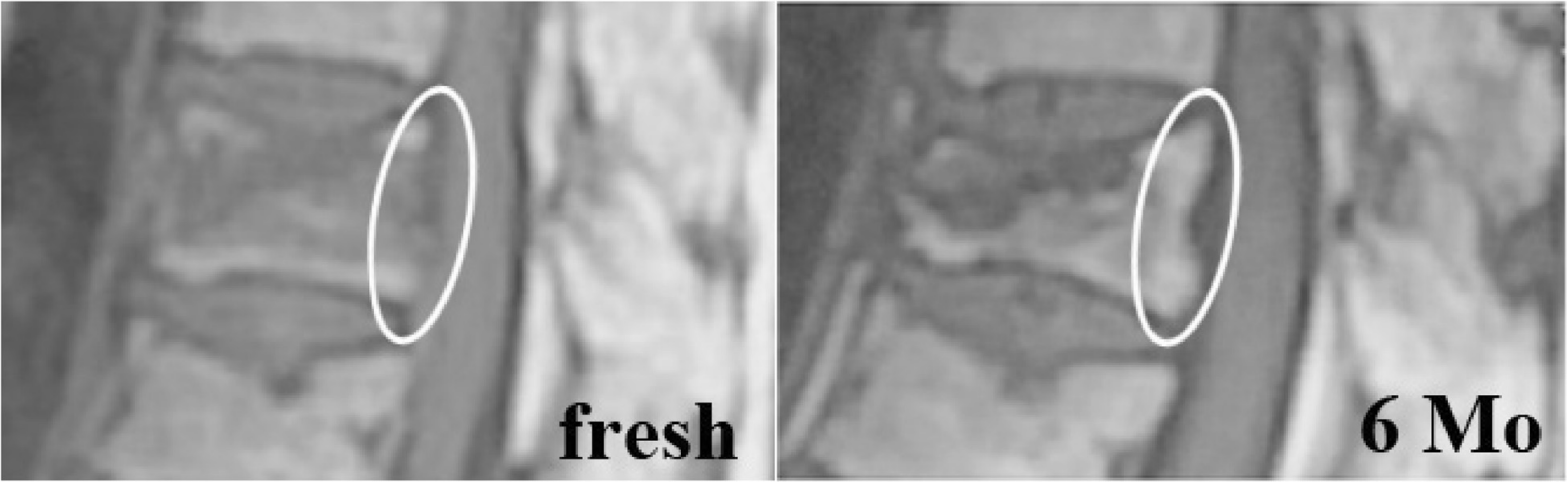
Representative image of chronological intensity change at posterior wall on T1-weighted magnetic resonance image. Intensity change at posterior wall was observed in the acute stage (left), but the intensity change was disappeared at 6 months (right).
